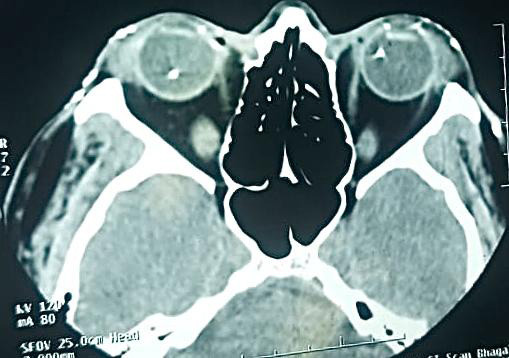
CT scan of the orbit showed multiple metallic densities in and around both orbits and bilateral maxillofacial soft tissue.
